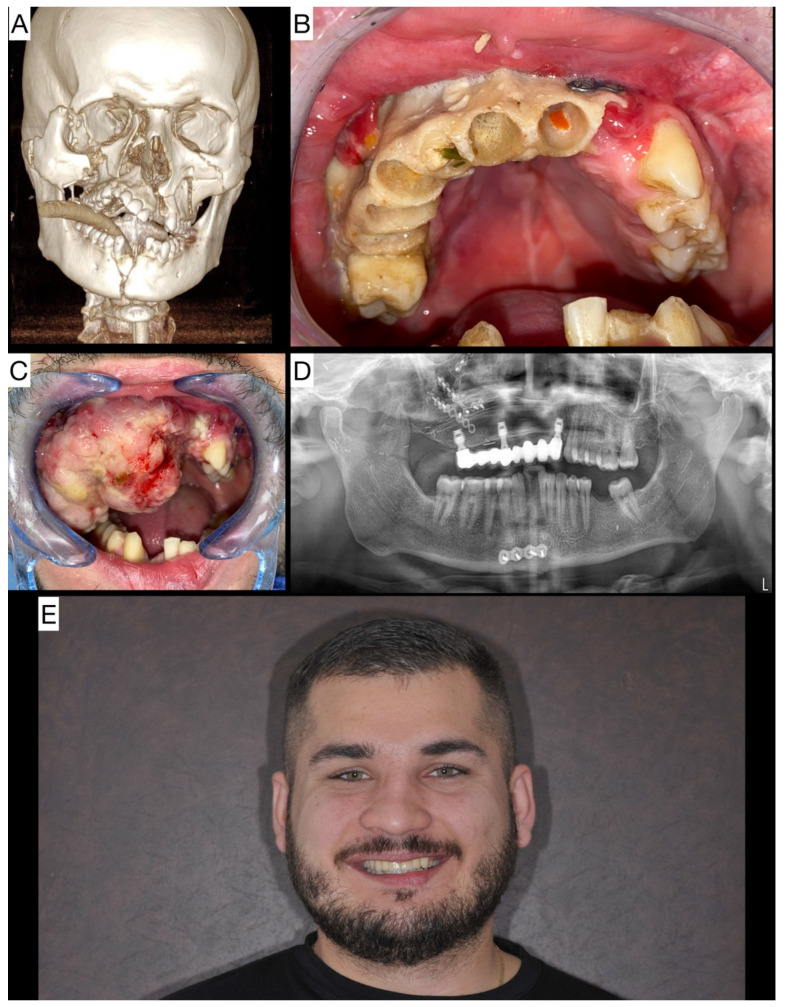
(A) 3D CT of panfacial trauma after a car accident. (B) Intraoral image of aseptic osteonecrosis of the maxilla, with demarcation of large bone segment from the surrounding healthy tissue. (C) Intraoral image after reconstruction of maxilla with DCIA and hyperplastic granulation of muscle tissue from the free flap. (D) OPG of final prosthesis supported by three extra short implants. (E) Image of the patient while smiling.
